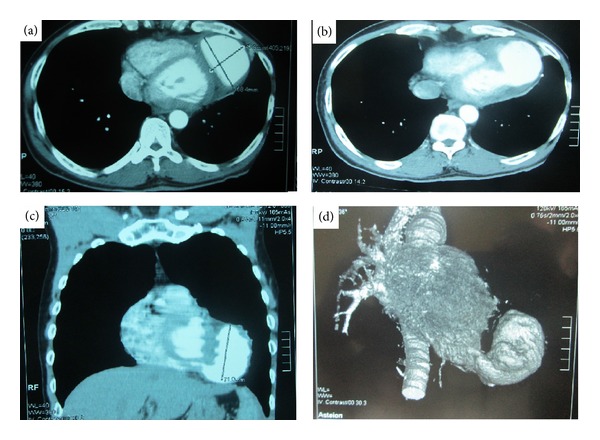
Chest CT scan imaging. (a) Cross-section showing the diameters of the pseudoaneurysm (45.9 mm × 68.4 mm). (b) Visualization of the neck between the left ventricle and the false aneurysm. (c) shows a sagittal diameter of 71 mm. (d) 3D reconstruction of the left ventricle communicating with the pseudoaneurysm.
